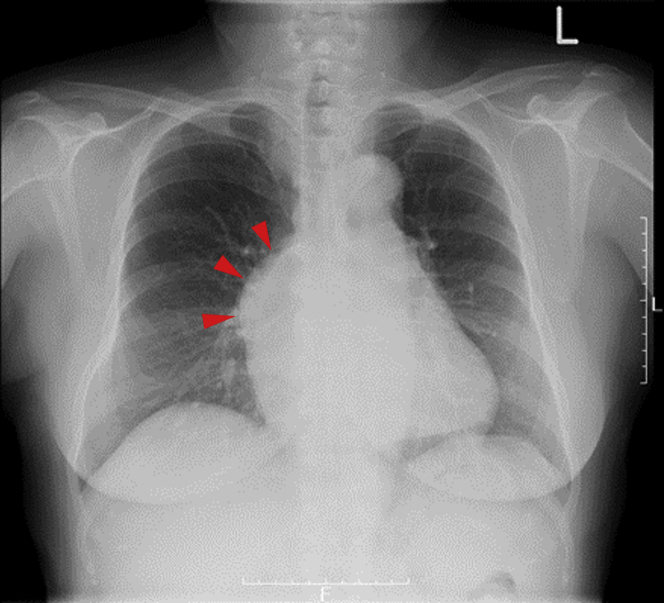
Pre-Operative Chest Radiography Abnormal shadow on the right side of the heart (red arrowheads).
